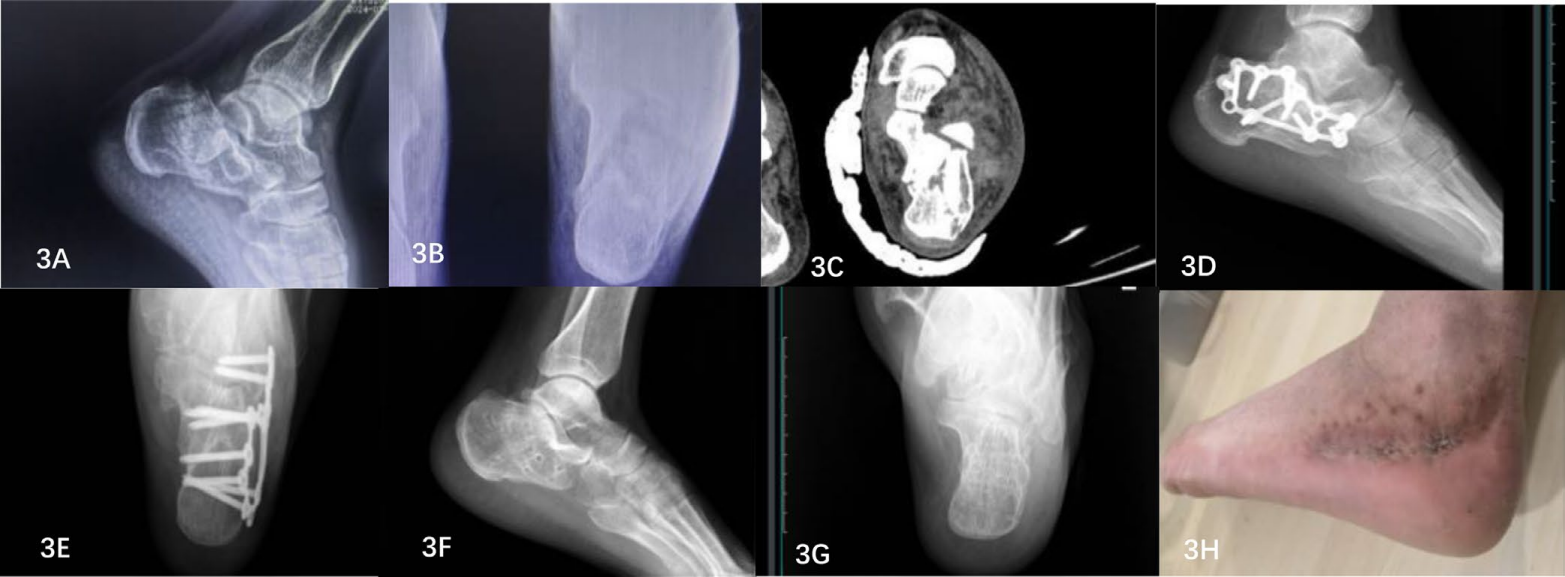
Imaging data of a typical ELIPIF patient. (A) Preoperative calcaneal lateral x‐ray image showing that the articular surface of the calcaneus collapsed. (B) Preoperative axial x‐ray of the calcaneus revealed that the width of the calcaneus increased and that the length of the calcaneus shortened. (C) Preoperative MR image of the calcaneal fracture suggested a Sander type III fracture. (D) Postoperative calcaneal lateral x‐ray image showing calcaneal height recovery. (E) Postoperative calcaneal axial x‐ray image showing that the width and length of the calcaneus had recovered. (F) Lateral x‐ray films of calcaneus after removal of internal fixator showed calcaneus recovery. (G) Axial x‐ray films of calcaneus after removal of internal fixator showed calcaneus recovery. (H) The wound healed well after the operation.
